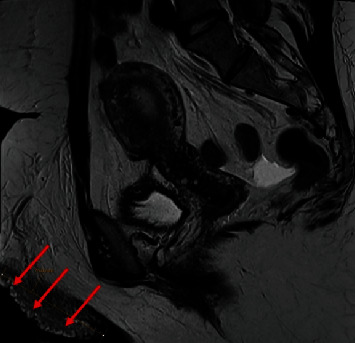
MRI pelvis sagittal T2 weighted image demonstrates 7.5 cm completely extra-pelvic hyperintense lobulated multicystic lesion (red arrow) in the left labia majora (vulvar lymphangioma).
